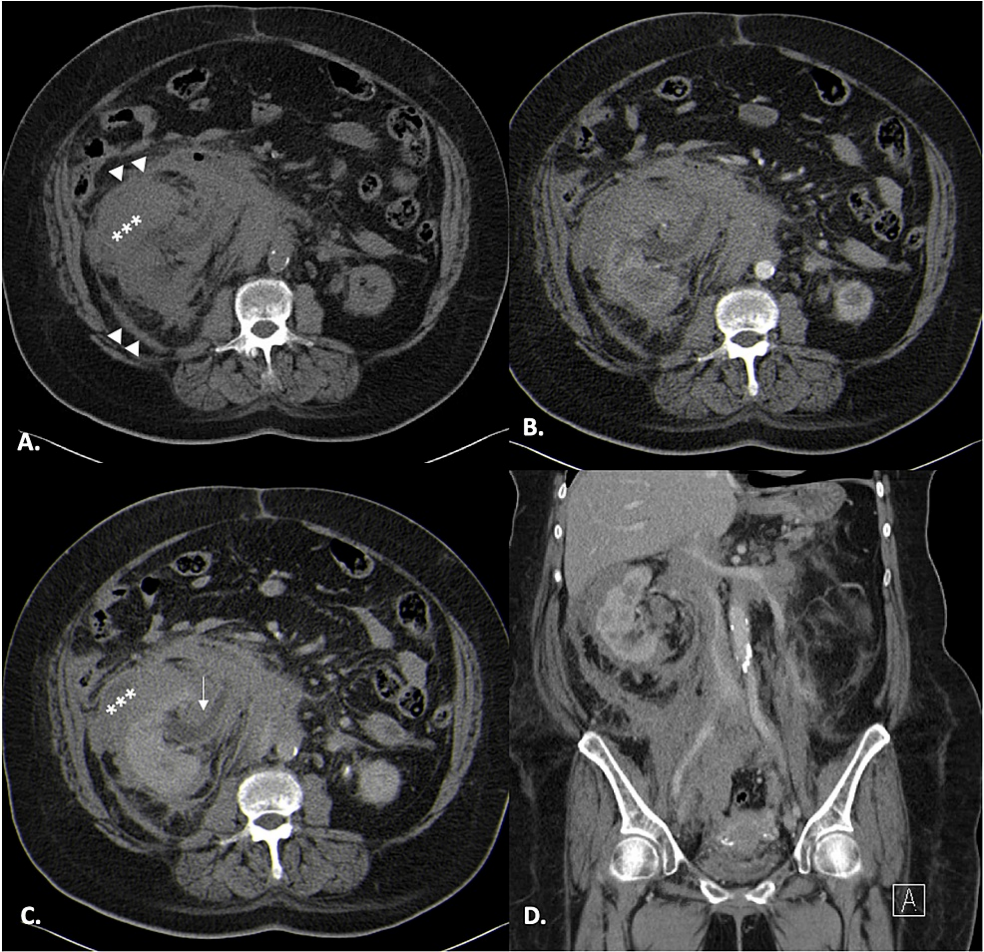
Initial CT scan of a 65-year-old female with primary renal lymphoma. Axial non-contrast CT (A) image of the right kidney depicts densification of the perirenal and anterior pararenal right spaces with mean of 35 HU (white asterisks in A and C); thickening of the anterior and posterior renal fasciae (white arrowheads in A). Axial arterial and portal phases (B and C) at the same level show slight enhancement, although less than the renal cortex (with mean HU of this densification in the arterial phase of 47 and in the portal phase 65). Coronal imaging at portal venous phase (D) depicts the vessels slightly molded but not invaded, and still permeable. Also, note associated right hydronephrosis (white arrow in C).
